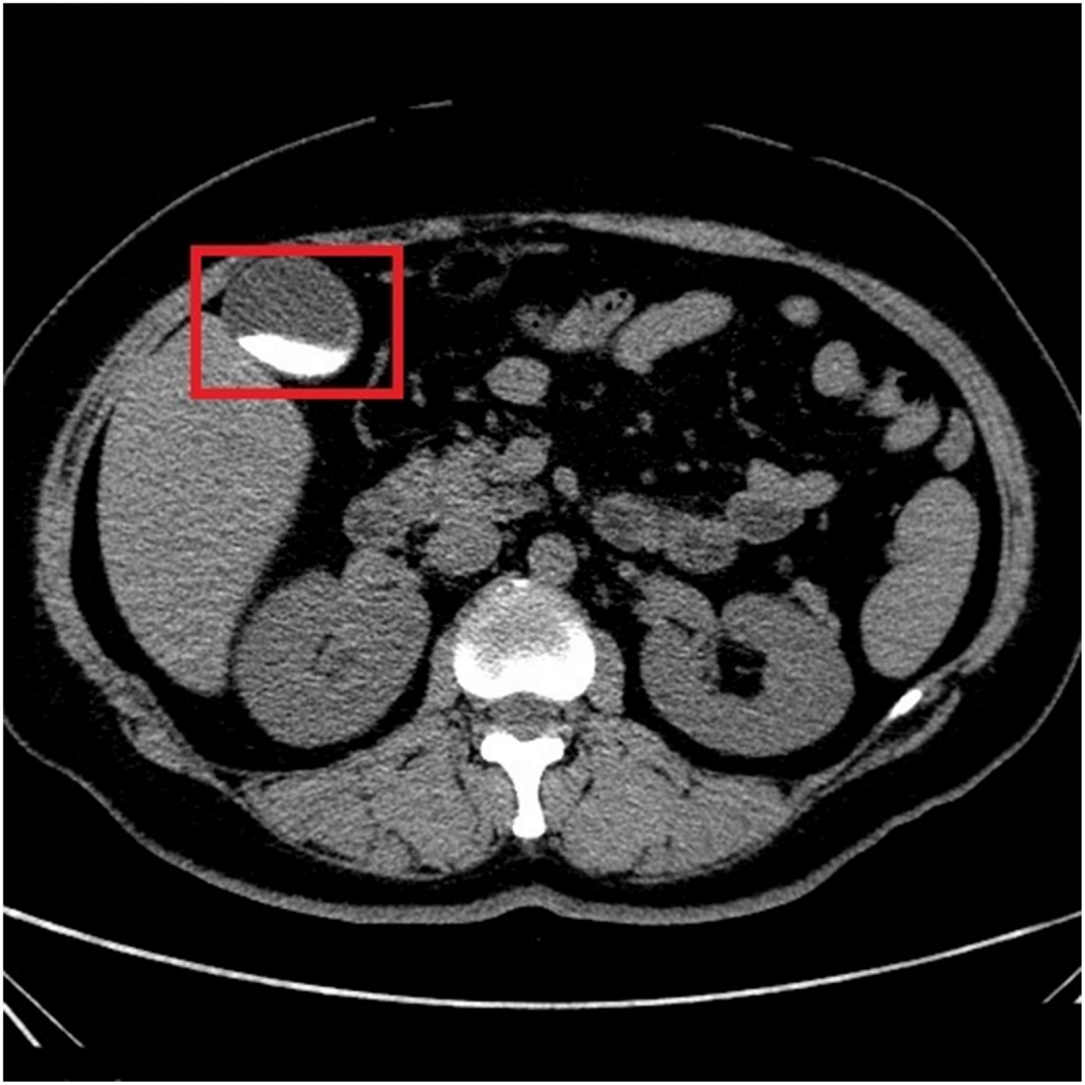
The CT image of a person has a higher degree of disease.
